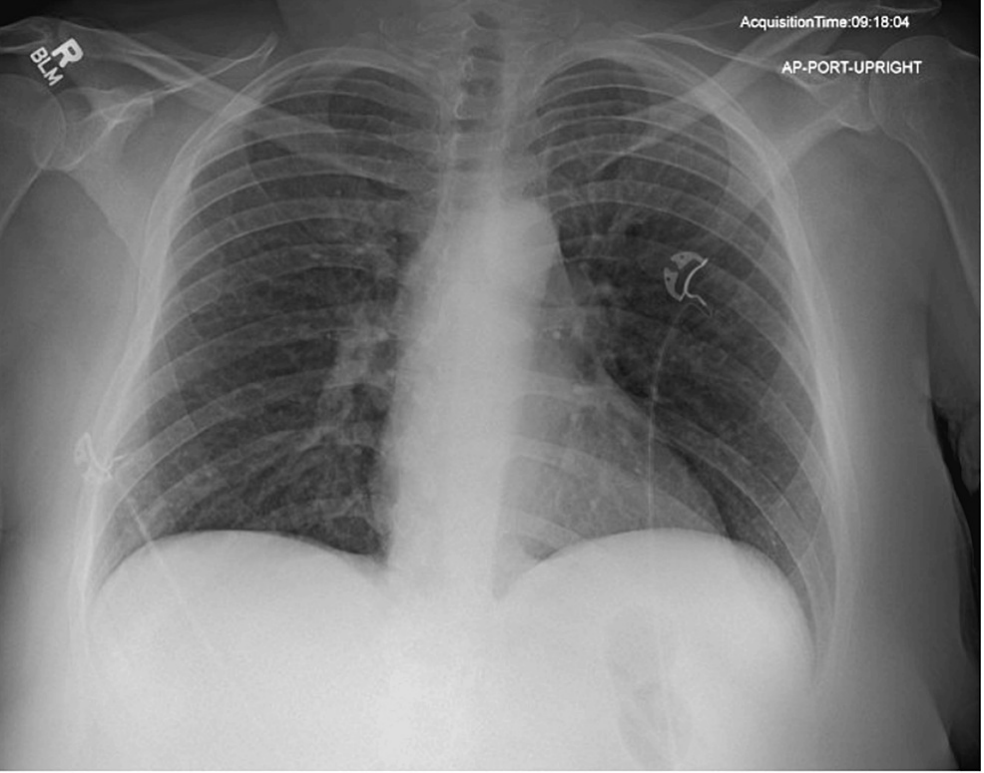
Chest X-ray on admission showing no acute cardiopulmonary process.
